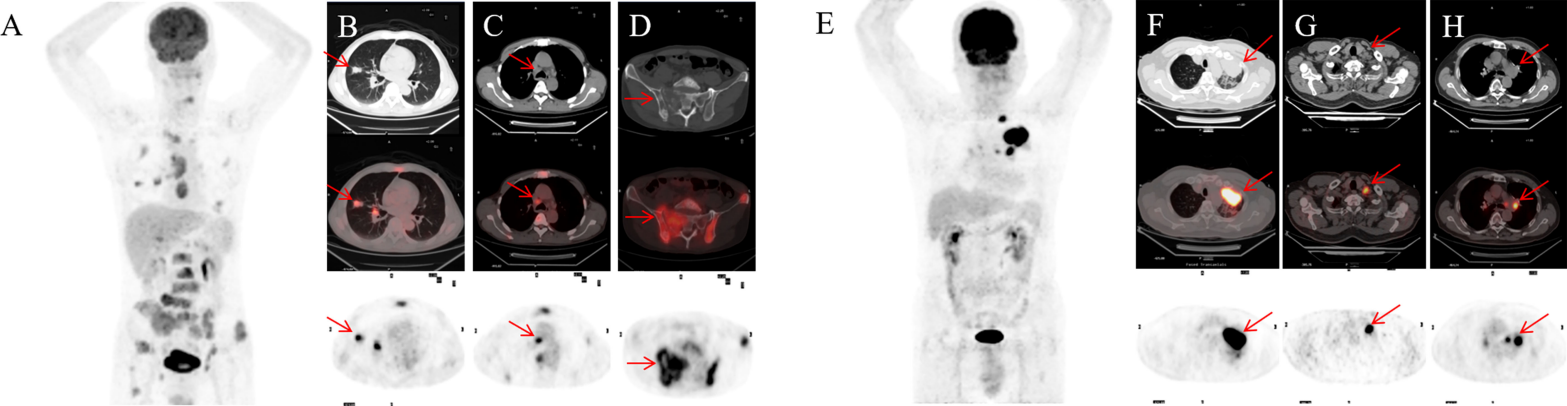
A 55-year-old male NSCLC patient with a history of smoking (A). Before treatment, 18F-FDG PET-CT revealed lesions in the right upper lobe and lymph node and pelvic bone metastases (B-D). After 4 months of targeted therapy, the patient died. A 67-year-old male NSCLC patient with a smoking history (E). Before treatment, 18F-FDG PET-CT found lesions in the upper lobe of the left lung, mediastinal and hilar lymph node metastasis (F-H). At 11 months after targeted therapy, the patient’s disease progresses.
